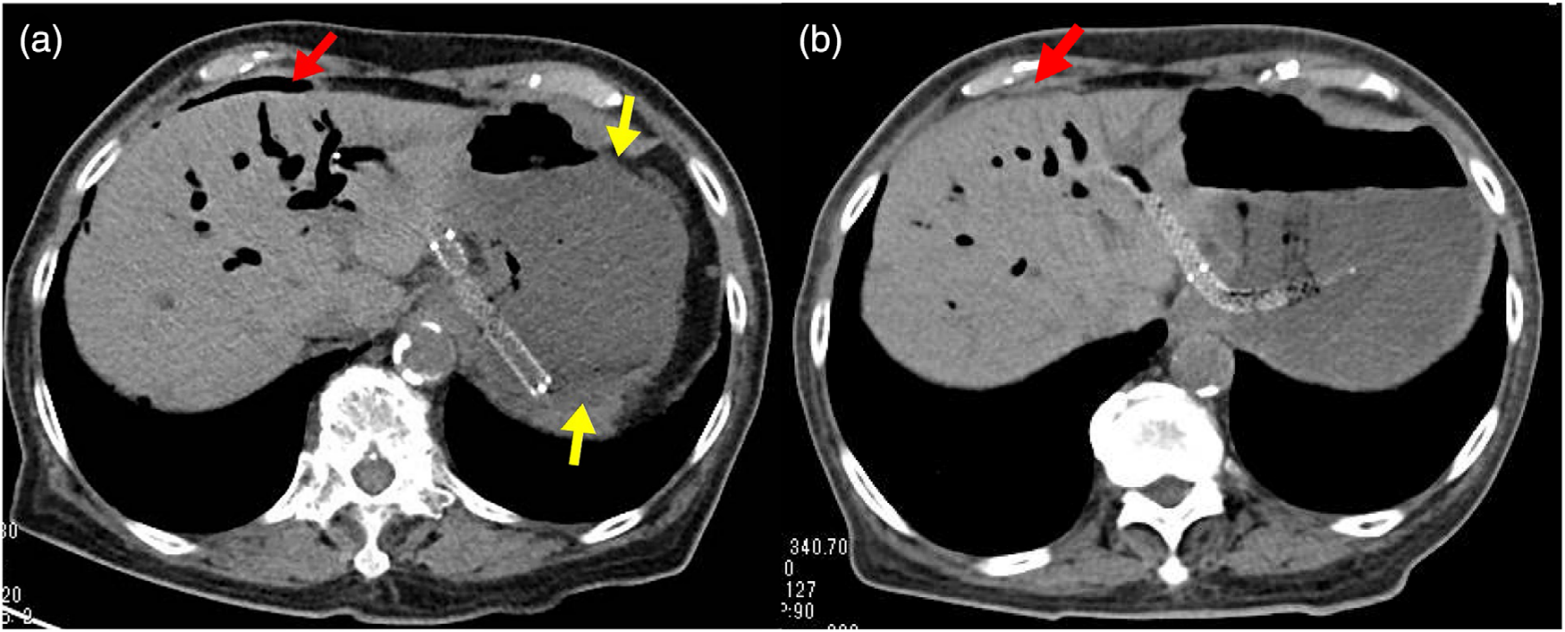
Comparison of the volume of free air between before and after the duodenal stent insertion by abdominal computed tomography. (a) When the patient complained of fever and abdominal pain, Abdominal computed tomography shows free air around the liver (red arrow) with food stored in the stomach (yellow arrow). (b) After the duodenal stent insertion, free air around the liver decreased (red arrow) compared with that before the procedure
